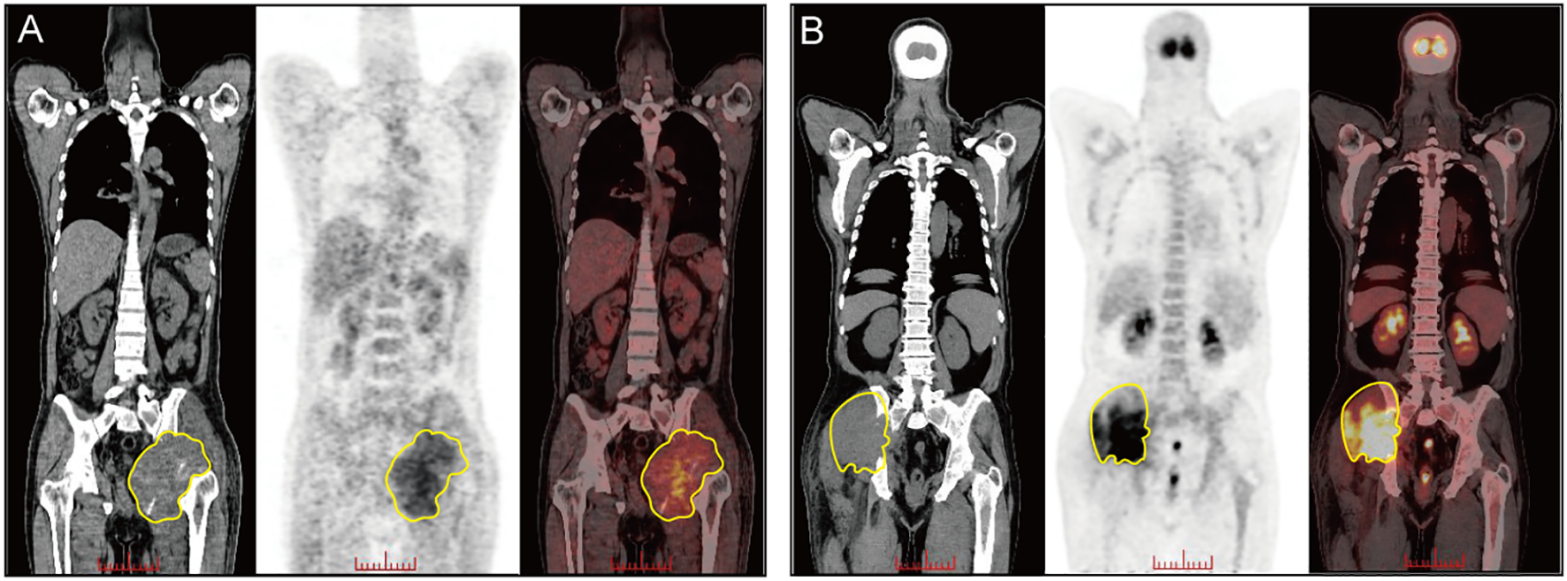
Positron emission tomography–computed tomography (PET-CT) images showing hypermetabolic osteolytic lesions with associated soft tissue masses: (A) Case 1, involving the left ischium and acetabulum; (B) Case 2, involving the right sacrum and adjacent pelvic bones.
